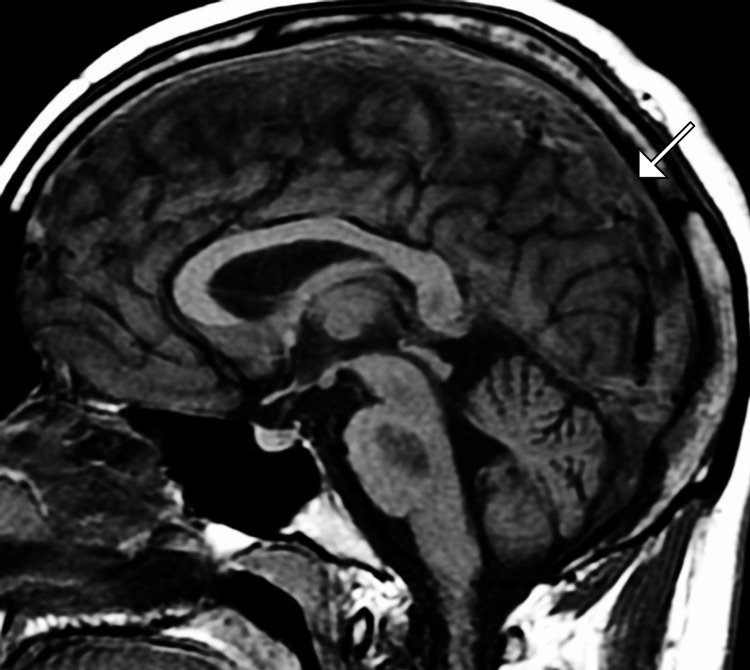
Sagittal MRI image shows an acute thrombus in the superior sagittal sinus (arrow) MRI: magnetic resonance imaging
